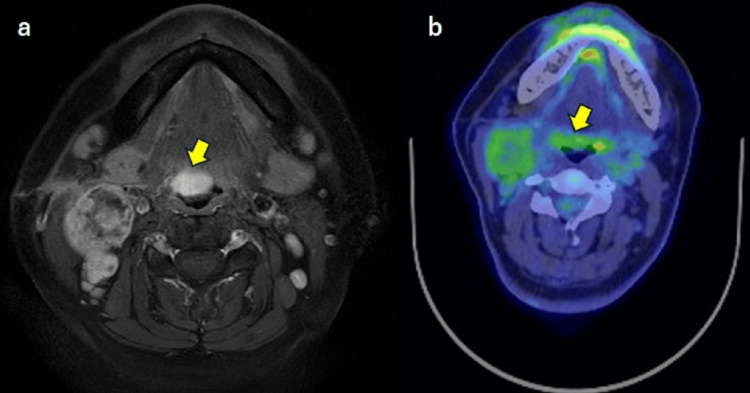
MRI axial view T1 with Gd enhancement and PET/CT axial view The arrow indicates a tumor on the base of the tongue and metastasis in bilateral cervical lymph nodes. PET/CT: Positron emissions tomography/computed tomography; Gd: Gadolinium
